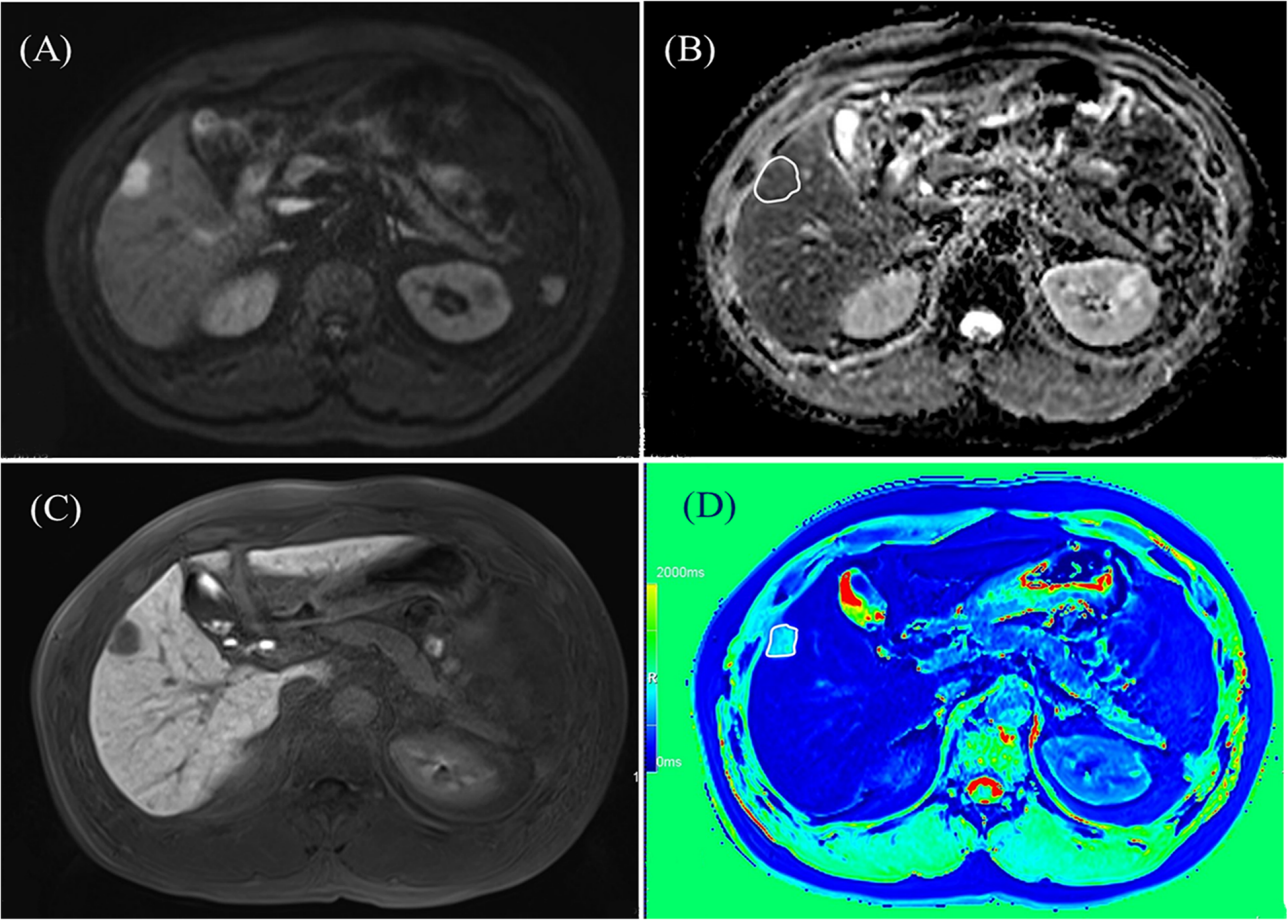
The ROIs of HCC was drawn on the corresponding ADC maps and postcontrast T1 maps. a the HCC on right lobe of liver showing hyperintensity on DWI maps of b = 500 mm^2^/s; b ROI was drawn on corresponding ADC maps; c the HCC on right lobe of liver showing hypointensity on hepatobiliary phase; d ROI was drawn on postcontrast T1 maps
